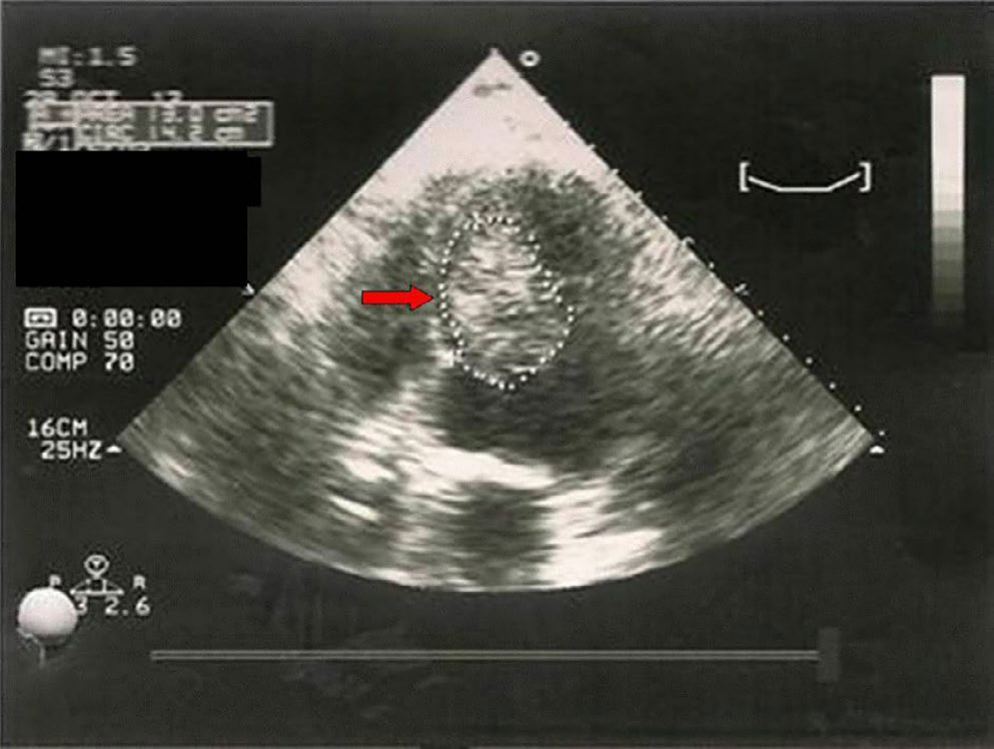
Preoperative TTE: Apical 4‐chamber view showing the tumor in the left chamber, attached to the septal wall (red arrow)
